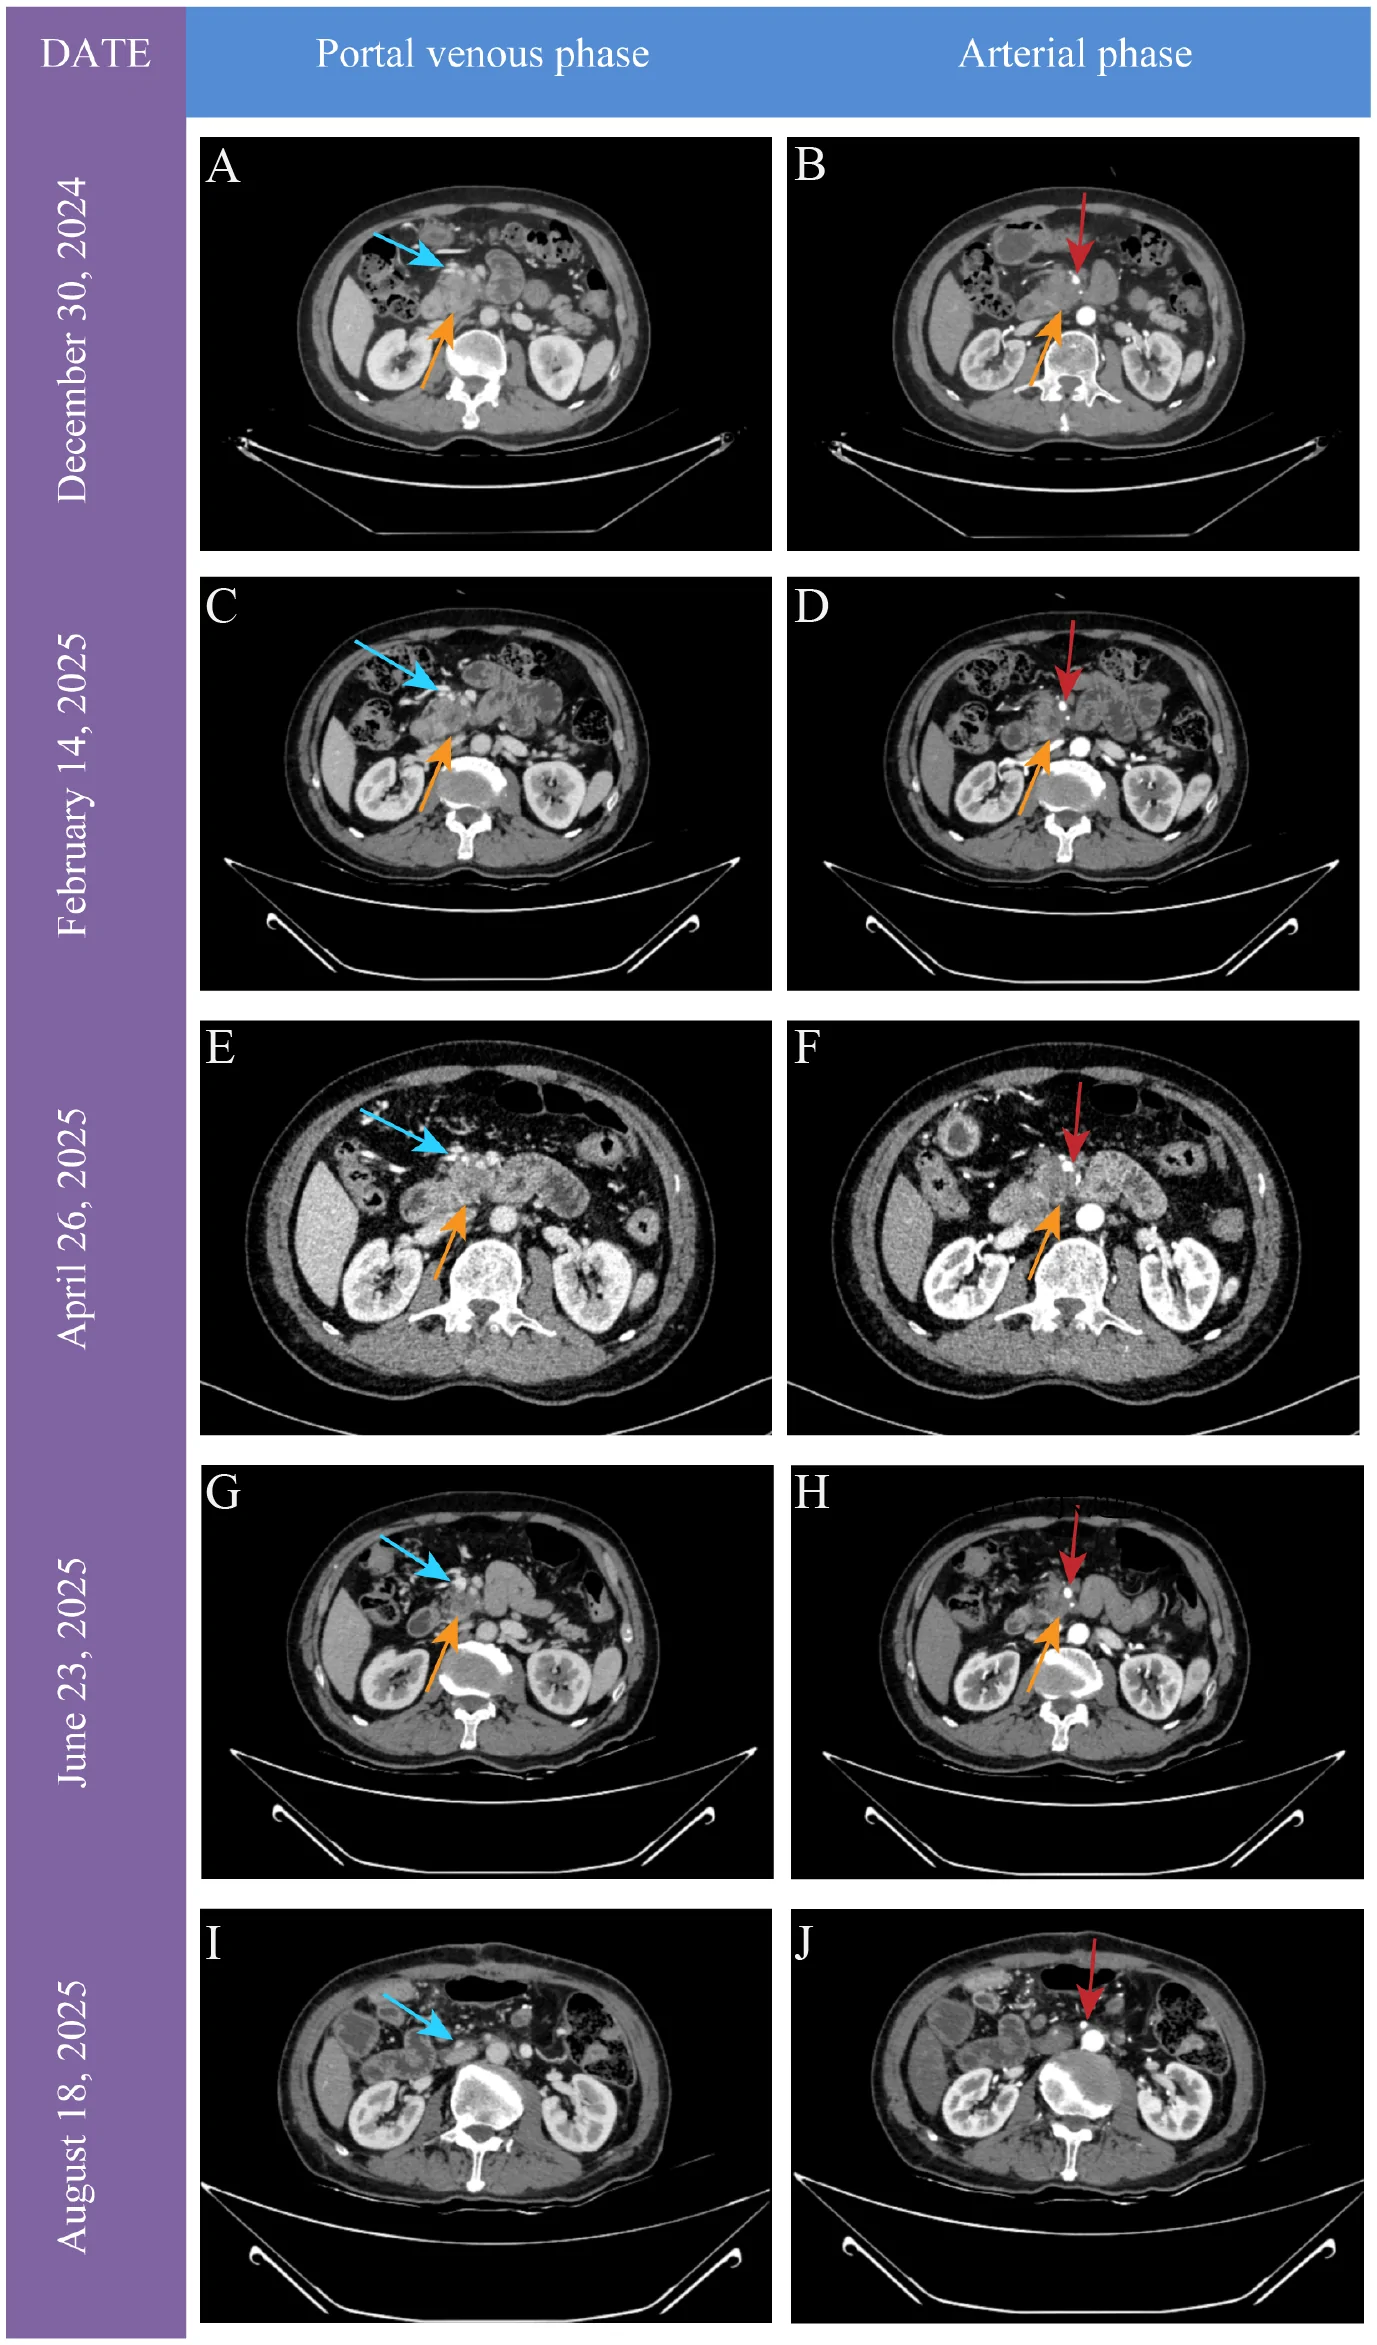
Serial contrast-enhanced CT images before and after surgery. (A–H) Four preoperative contrast-enhanced CT examinations (arterial and portal venous phases) demonstrated a marked reduction in the size of the primary tumor and alleviation of vascular involvement, including the SMV and SMA, following neoadjuvant therapy. The tumor is indicated by yellow arrows, SMV involvement by blue arrows, and SMA involvement by red arrows. (I, J) One postoperative follow-up contrast-enhanced CT scan showed no abnormal enhancement or enlarged lymph nodes after radical pancreaticoduodenectomy.
